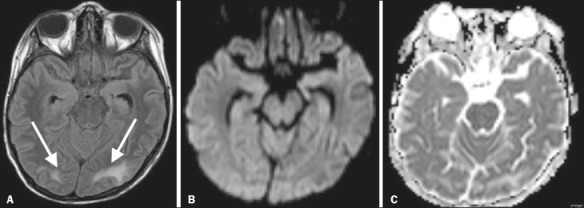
Brain MRI. Axial T2-weighted FLAIR sequence (A) showing lesions in typical
